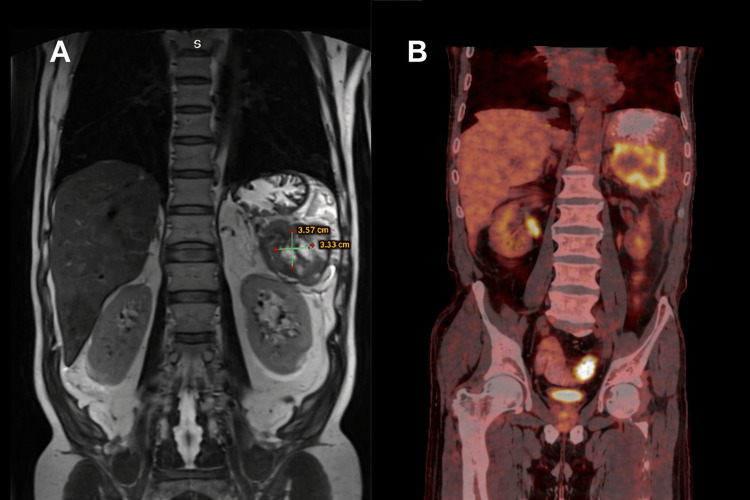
Preoperative radiological imaging A: T2-weighted coronal MRI showing a heterogeneous mass in the projection of the pancreatic tail (measured at 3.57 x 3.33 cm in this plane). B: PET/CT scan demonstrating intense fluorodeoxyglucose (FDG)-avidity (pathological contrast uptake) in the pancreatic tail lesion, consistent with metastatic involvement.
